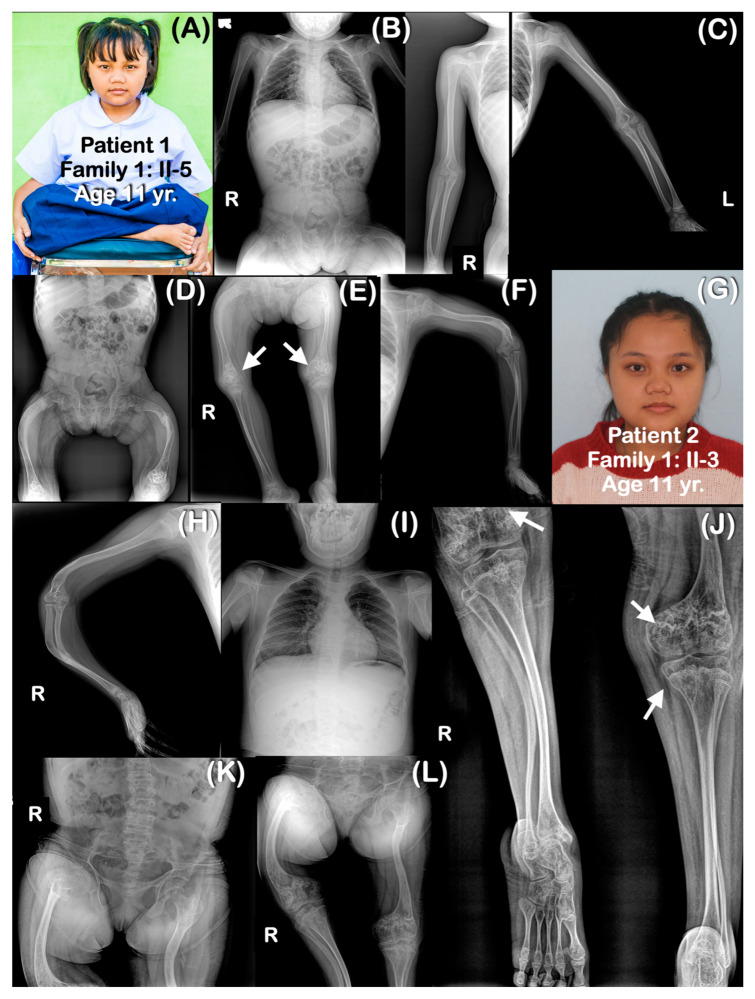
Family 1. (A–F) Patient 1 (Family 1: II-3). Age 11 years. Generalized osteopenia is noted. Ribbon rib deformity is observed. (E) Popcorn calcifications are observed at distal femoral metaphyses (arrows). Femora are shortened and curved. Fracture of left femur is healed. Humeri, radii, ulnae, tibiae, and fibulae are not bowed. (G–L) Patient 2 (Family 1: II-5). (G) Sister of patient 1. Age 17 years. Radiographs taken at 10 years. Generalized osteopenia is noted. Curved and slender long bones. The right femur is shorter than the left one. (L) Popcorn calcifications are observed at distal femora (arrows).
